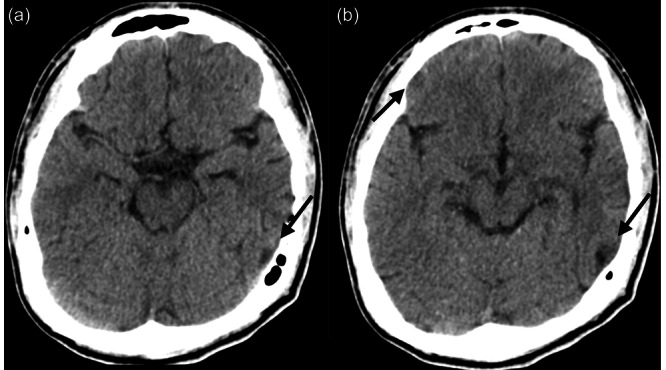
Brain computed tomography 5 months after the accident reveals known cerebral contusions in the right orbitofrontal cortex and left temporal lobe.
